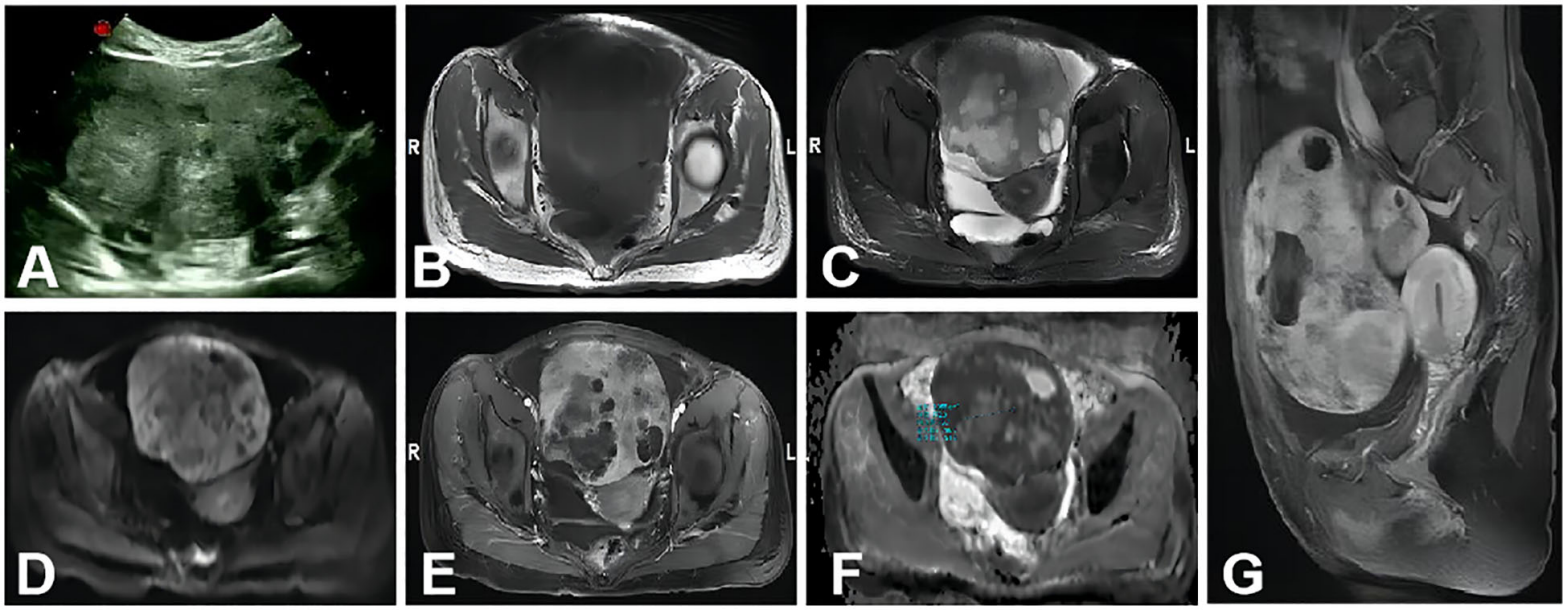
A 34-year-old woman with metastasis of gastric cancer to the bilateral ovaries. The large mass in the abdominal-pelvic cavity was from the right ovary. (A) A non-homogeneous middle-hypoechoic solid mass was detected by ultrasound examination. (B–D) A solid mass with clear border and necrosis shows mainly isointense, hyperintense, and hyperintense signal intensities on axial T1WI, T2WI, and DWI (b=1,000s/mm^2^), respectively. (E) Axial contrast-enhanced T1WI showed the solid portion was intensive enhancement. (F) On the ADC map, the mean ADC value was 0.772 × 10^−3^ mm^2^/s. (G) Sagittal contrast-enhanced T1WI showed that the mass was similar to (E).
